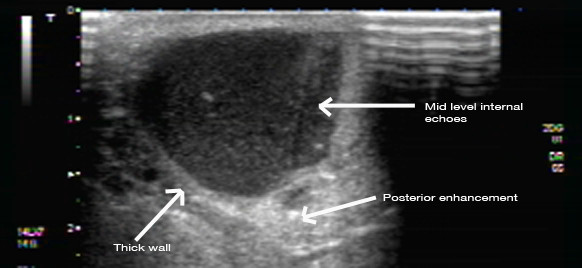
Ultrasound image of a palpable lump behind the nipple of a pregnant woman. The mass is thick walled with mid-level internal echoes and posterior enhancement.
